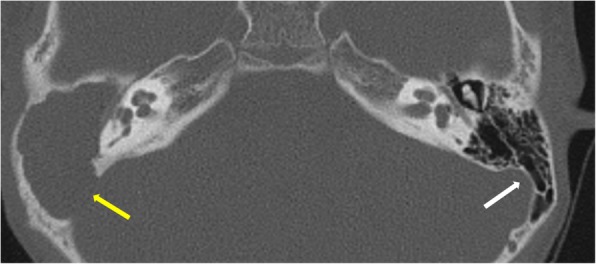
Axial CT shows normal “ice cream cone” configuration of ossicles in epitympanum on the left side formed by the head of the malleus and the body of the incus. The right ear shows soft tissue in the middle ear and aditus with non-visualization of ice cream cone suggesting erosion. A large defect is also seen in the sinodural plate on the right side (yellow arrow). Left sinodural plate is intact (white arrow)
